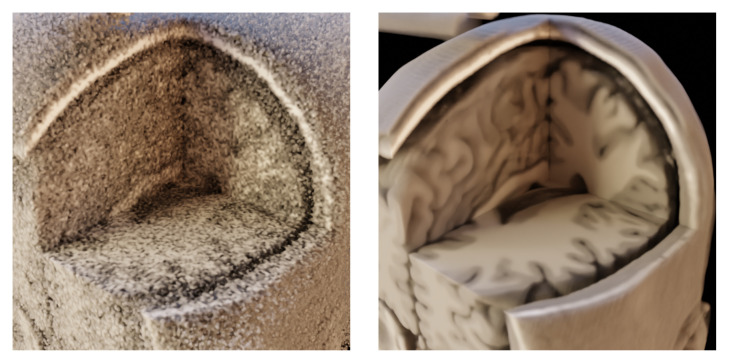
Volume rendering of MRI dataset from BrainWeb; (left)—the dataset corrupted by 25% Gaussian noise; (right)—the dataset without the noise; identical setup of a transfer function in shader properties for both data.
